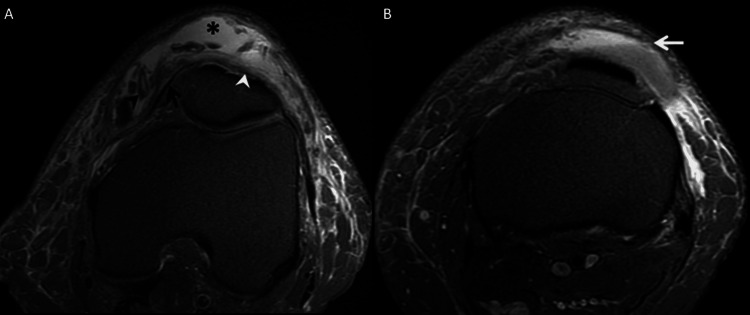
(A) Axial view of PD-FS MRI sequences demonstrates a hyperintense prepatellar fluid collection (asterisk) containing hemorrhagic debris (black arrowhead) and featuring a well-defined capsule (black arrow). The longitudinal fibers of the rectus femoris tendon extend behind the collection (white arrowhead). (B) Axial slice of a DP-FS sequence showing a blood-fluid level (white arrow). PD-FS, proton density fat-saturated; MRI, magnetic resonance imaging
